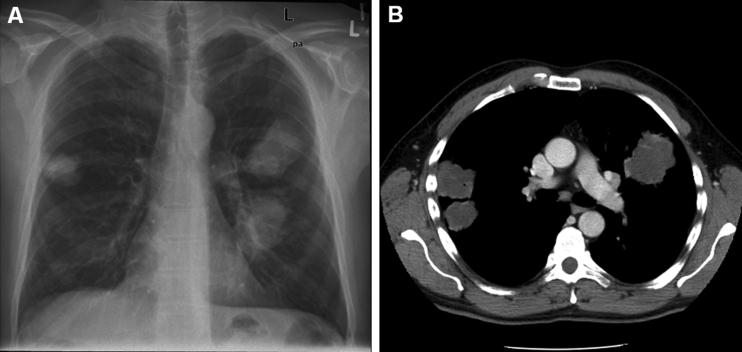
A) Chest radiograph and B) computed tomography scan of the patient showing 3 nodular *Cryptococcus gattii* infiltrates near pleura.
